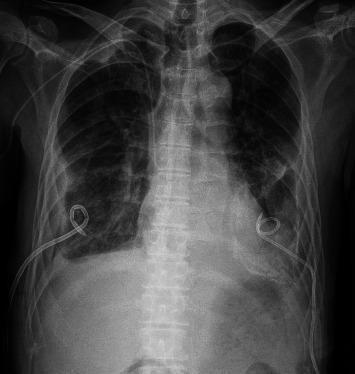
Chest x-ray prior to discharge after the patient's 2^nd^ relapse, demonstrating the interval improvement of bilateral pleural effusions.
